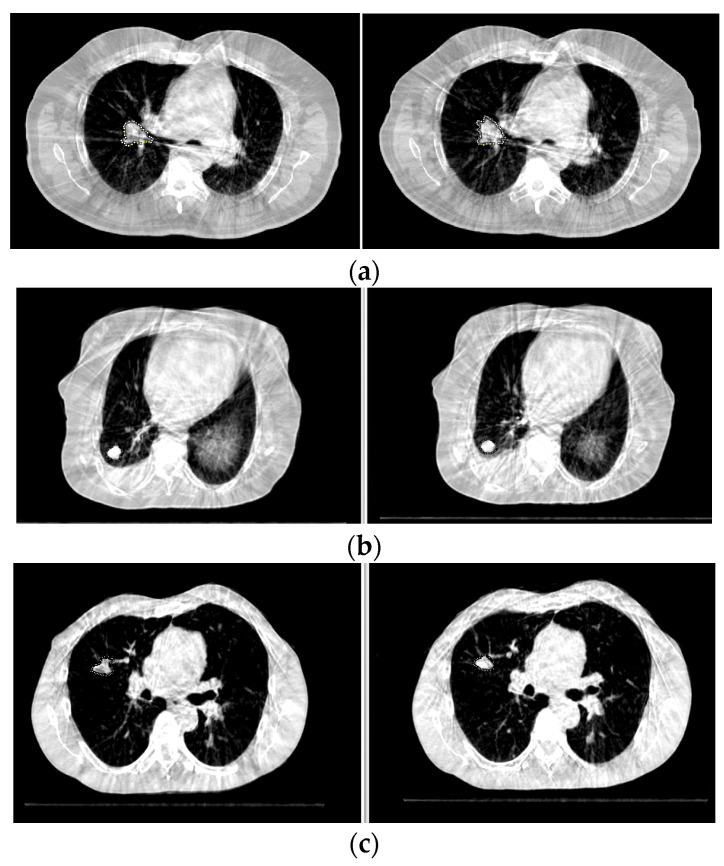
Tumor change between CBCT 1 (left) and CBCT 5 (right). *R* is consistent with RA, but *R_A_*, *R_CNR_*, and *R_μ_* are not consistent with RA. (a) Progression; (b) response; (c) stable. Tumors are contoured by an iso-pixel line.
